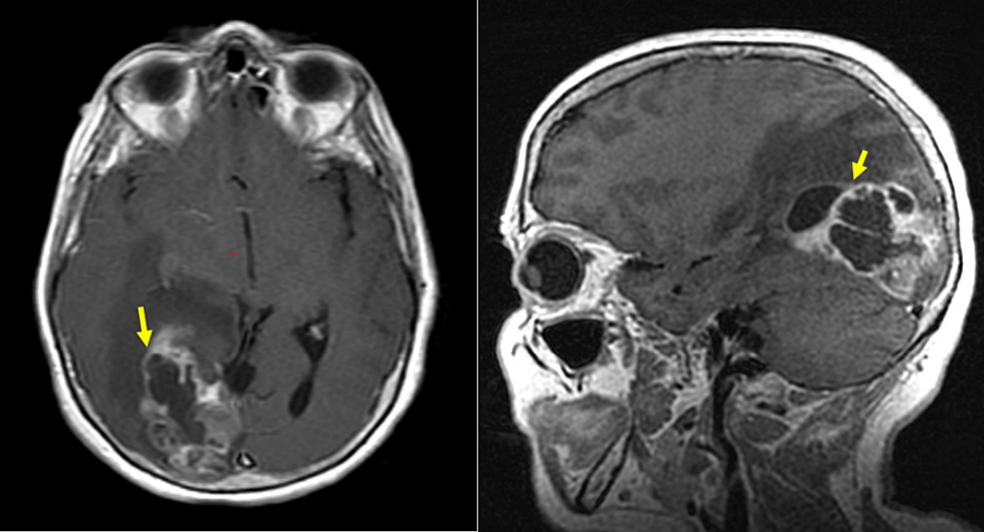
Initial MRI axial T1 SE post-contrast and sagittal T1 post-contrast of the head shows a peripherally enhanced complex mass with areas of necrosis in the parietotemporal and occipital regions, measuring approximately 6.4 x 3.2cm [yellow arrow]. There is a minimal leftward midline shift measuring roughly 8mm.
